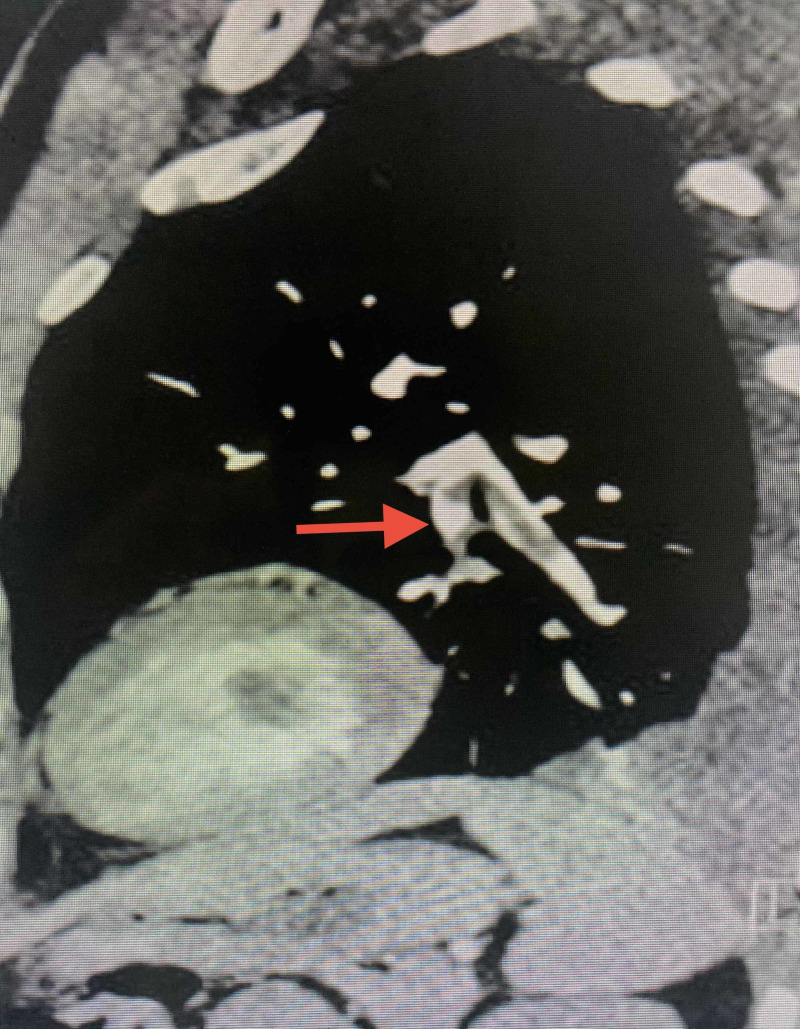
Sagittal slice of the computed tomography pulmonary angiography showing segmental and subsegmental filling defects in the left pulmonary artery.
